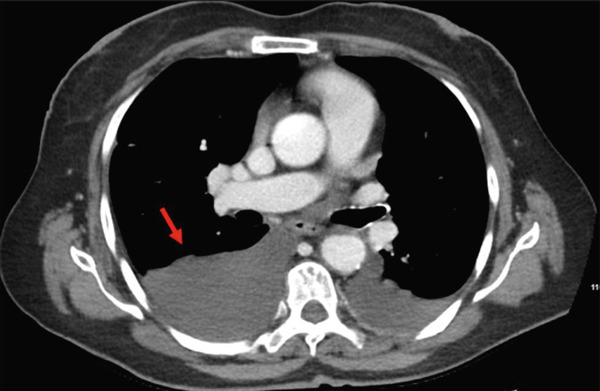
Bilateral pleural effusions on contrast‐enhanced CT of the chest, found incidentally and later confirmed to be chylous.
